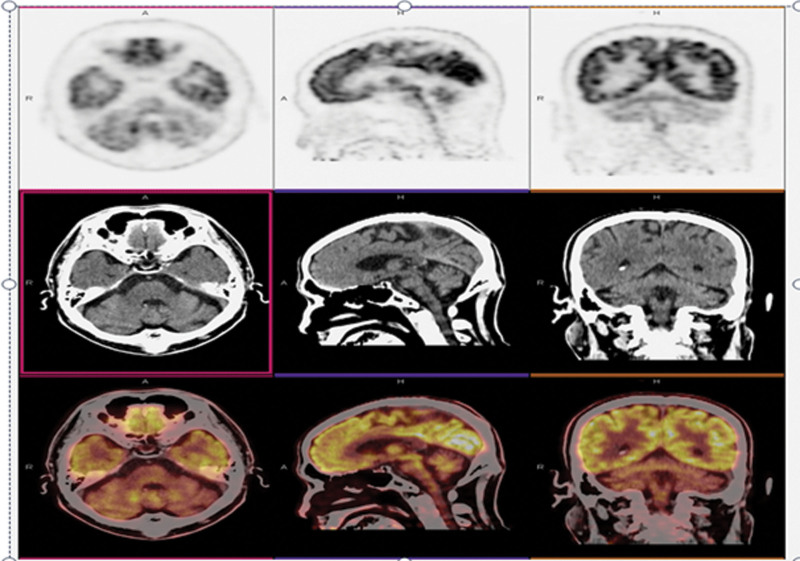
PET-CT showed diffuse slightly reduced metabolism in bilateral cerebellar hemispheres, the reduced volume of the Brainstem and cerebellum. PET-CT = positron emission tomography-computed tomography.
